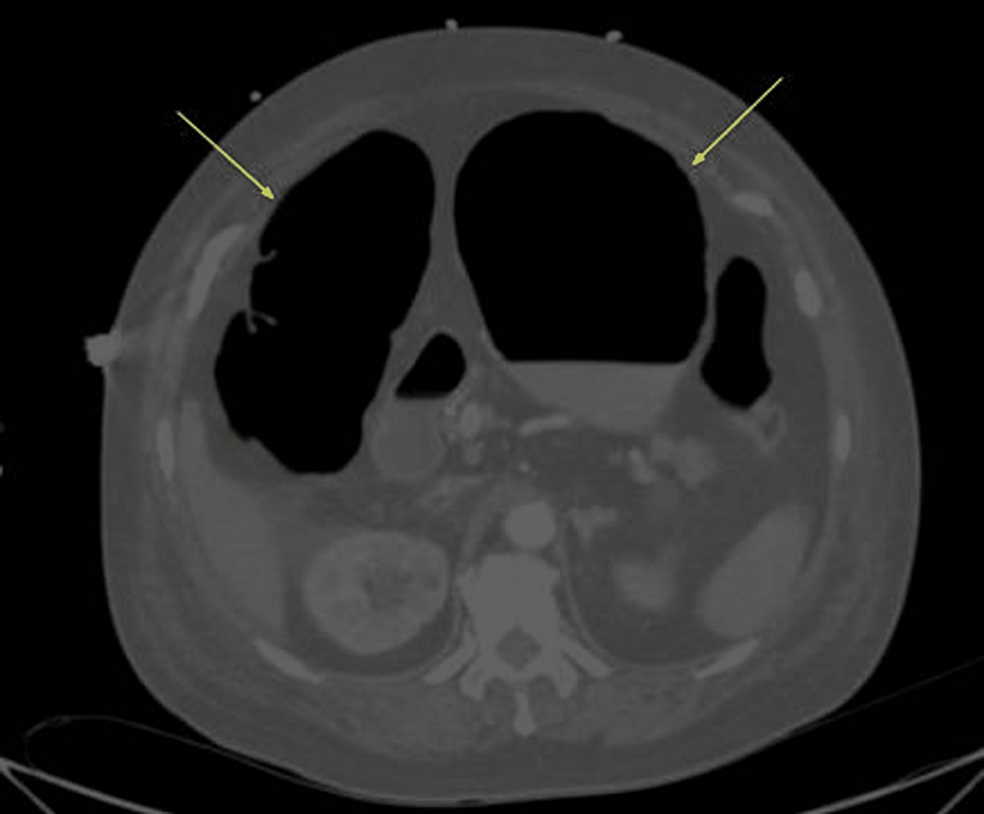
Abdominal CT scan axial image shows a 10 cm dilatation involving the ascending and transverse colon (yellow arrows)
